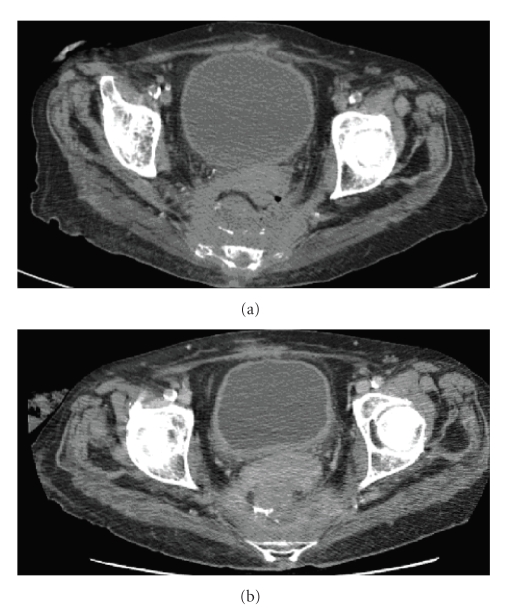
(a) CT precystoscopic tack removal. Note the thick
